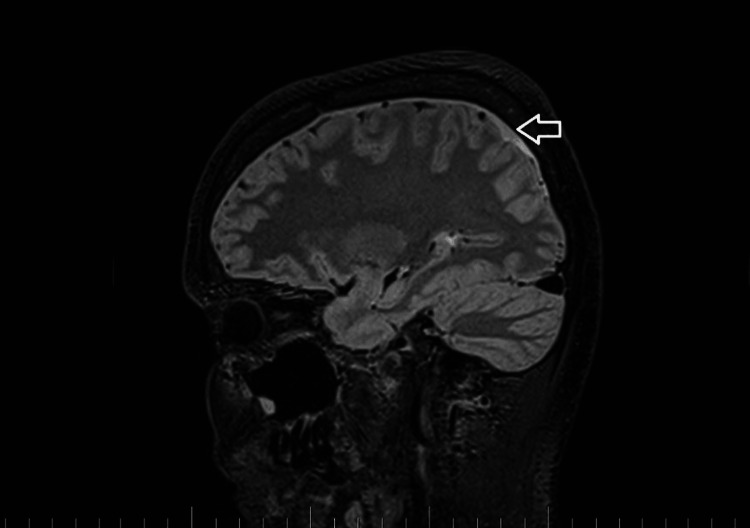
Brain MRI on March 5, 2023. Sagittal T2-weighted images. The white arrow shows a regression of the subdural fluid collection in the parietal area to 2.5 mm.
